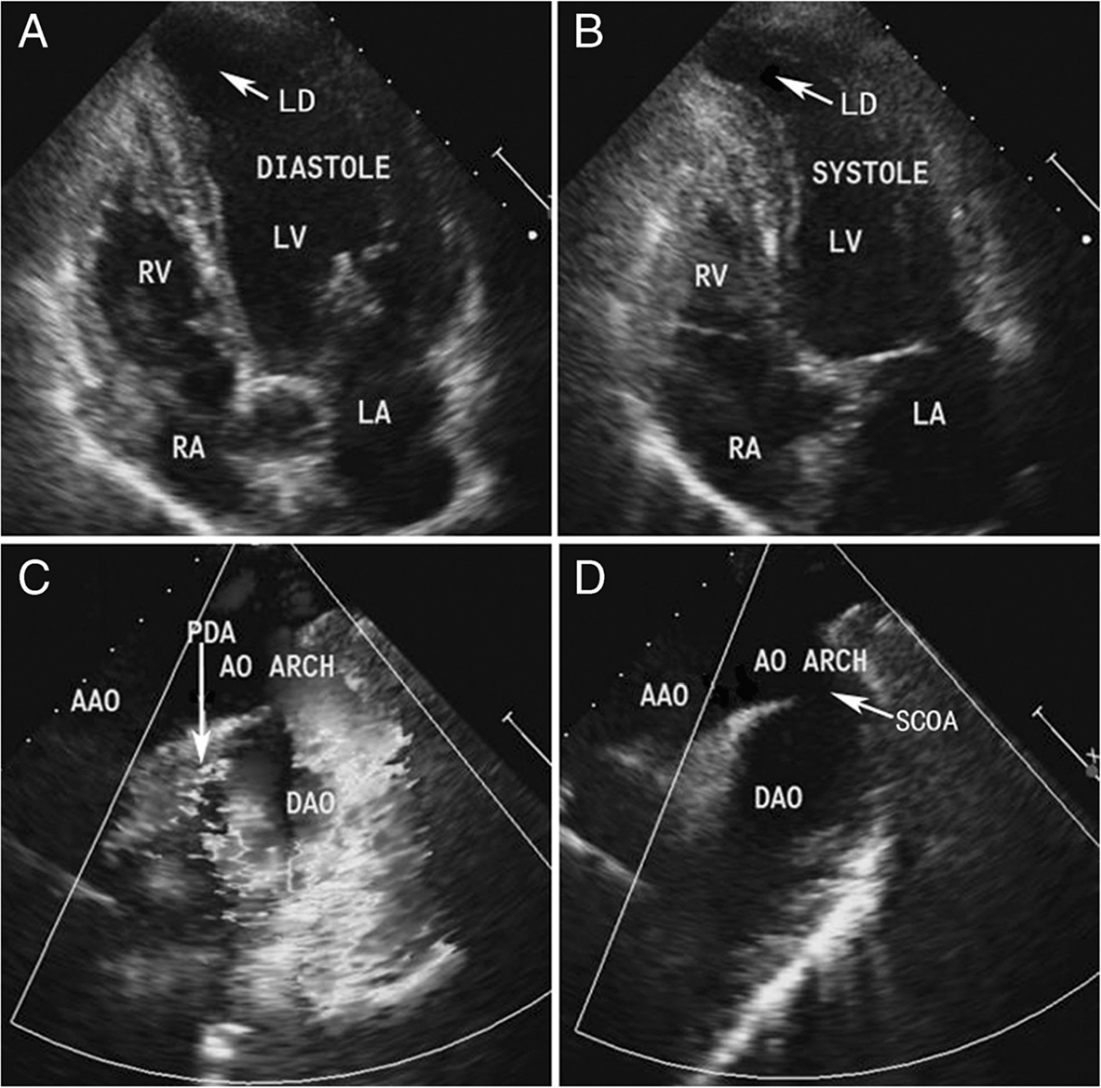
Echocardiogram showing a left-ventricular diverticulum (LD), a PDA and a suspicious coarctation of the aorta (SCOA) (a to d). LV, left ventricle; RV, right ventricle; LA, left atrium; RA, right atrium; AAO, ascending aorta; AO ARCH, aortic arch; DAO, descending aorta
